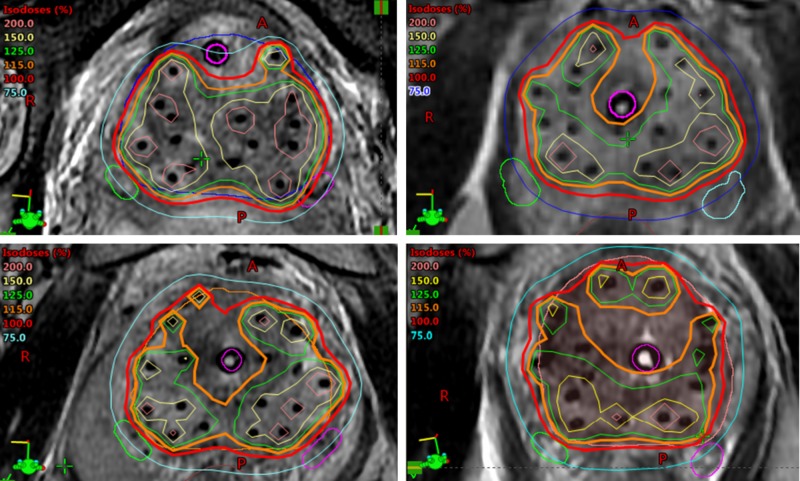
Examples of HDR brachytherapy dosimetry Four (upper left, upper right, lower left, lower right) representative patient examples of MRI-based HDR brachytherapy dosimetry in the axial plane. Note contours of neurovascular bundles at posterior-lateral aspects of prostate gland. MRI: magnetic resonance imaging; HDR: high dose rate
